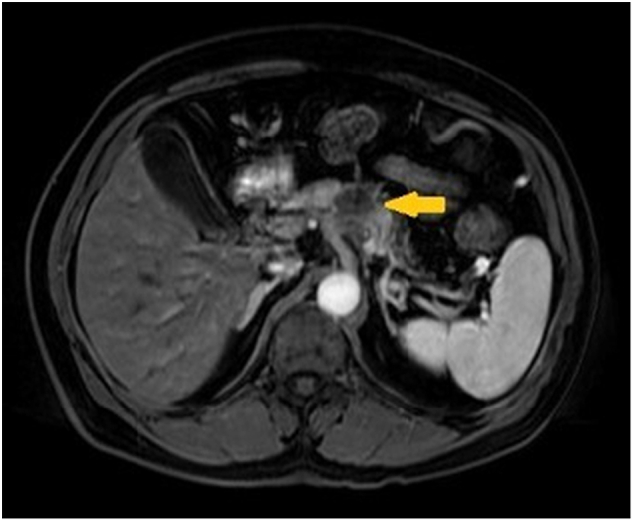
CT image after neoadjuvant chemotherapy. Small tumor reduction (indicated by the yellow arrow) measuring 3.0 × 2.6 cm. (For interpretation of the references to colour in this figure legend, the reader is referred to the web version of this article.)
